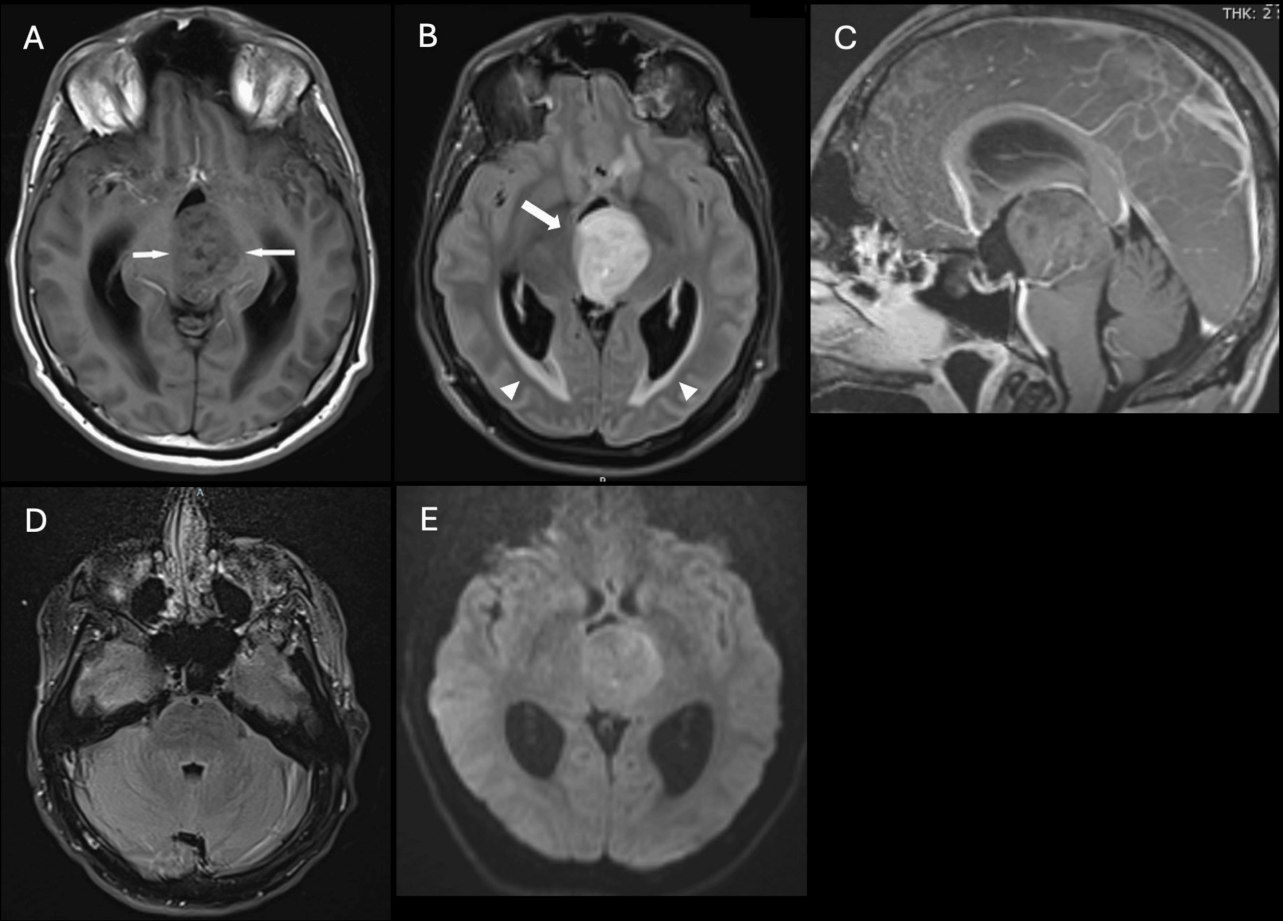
Tumor baseline MRI MRI of the midline mass measuring 3.3 × 3.7 × 3.1 cm at index hospitalization. (A) Axial non-contrast T1W image shows an intermediate signal, heterogeneous third ventricular mass (arrows). (B) Axial FLAIR image shows homogeneous T2 hyperintensity (arrow). Note transependymal CSF migration surrounding the occipital horns related to obstructive hydrocephalus (arrowheads). (C) Sagittal gadolinium-enhanced image shows minimal enhancement of the mass, occupying the third ventricle and invading the thalamus and mesencephalon. (D) Axial FLAIR image of the pons shows no tumor involvement. (E) Diffusion-weighted images demonstrated no significant restricted diffusion. MRI: magnetic resonance imaging, FLAIR: fluid-attenuated inversion recovery, CSF: cerebrospinal fluid
